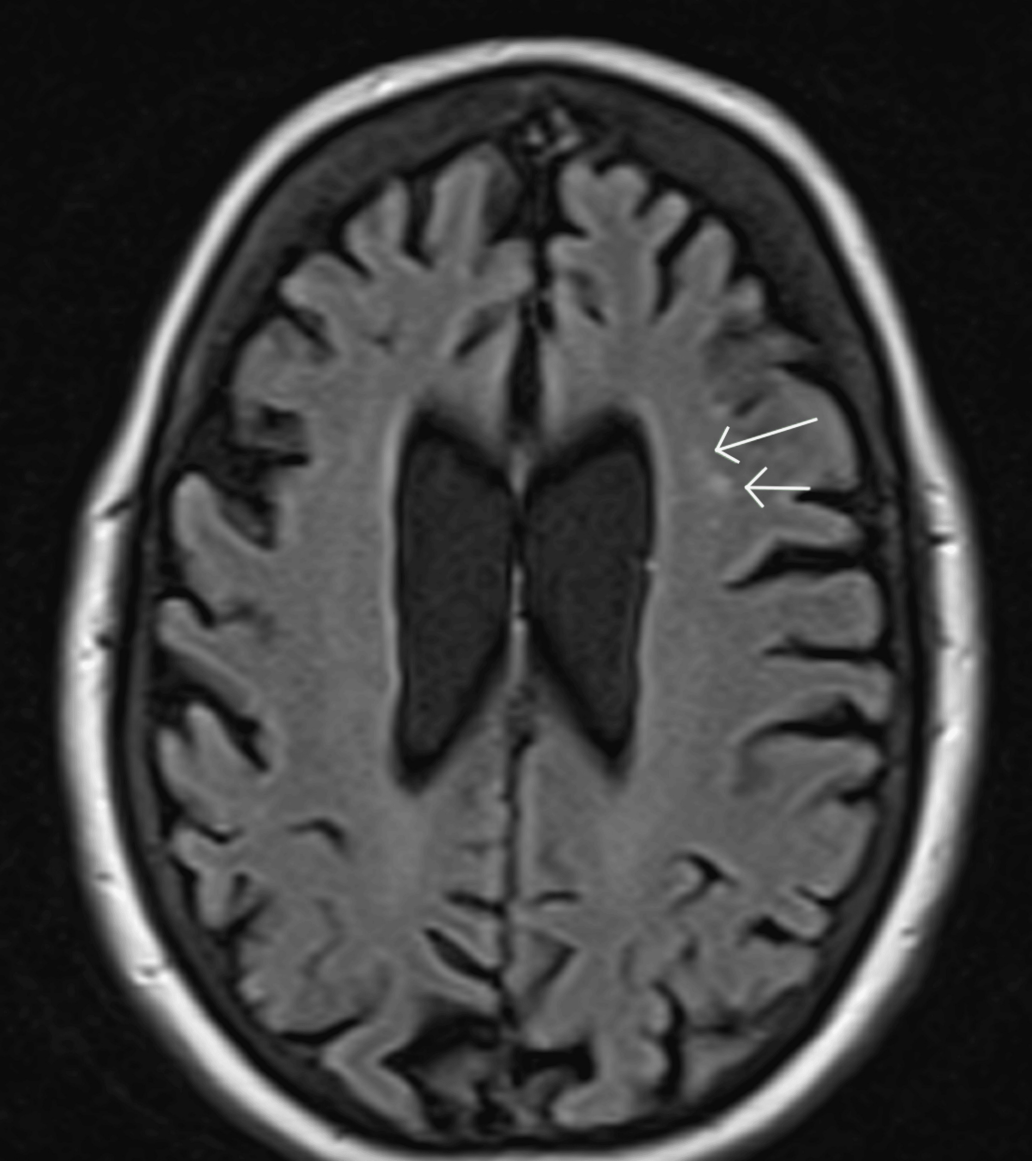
MRI showing small nonspecific T2-weighted hyperintensity in the left frontal lobe (white arrows).
